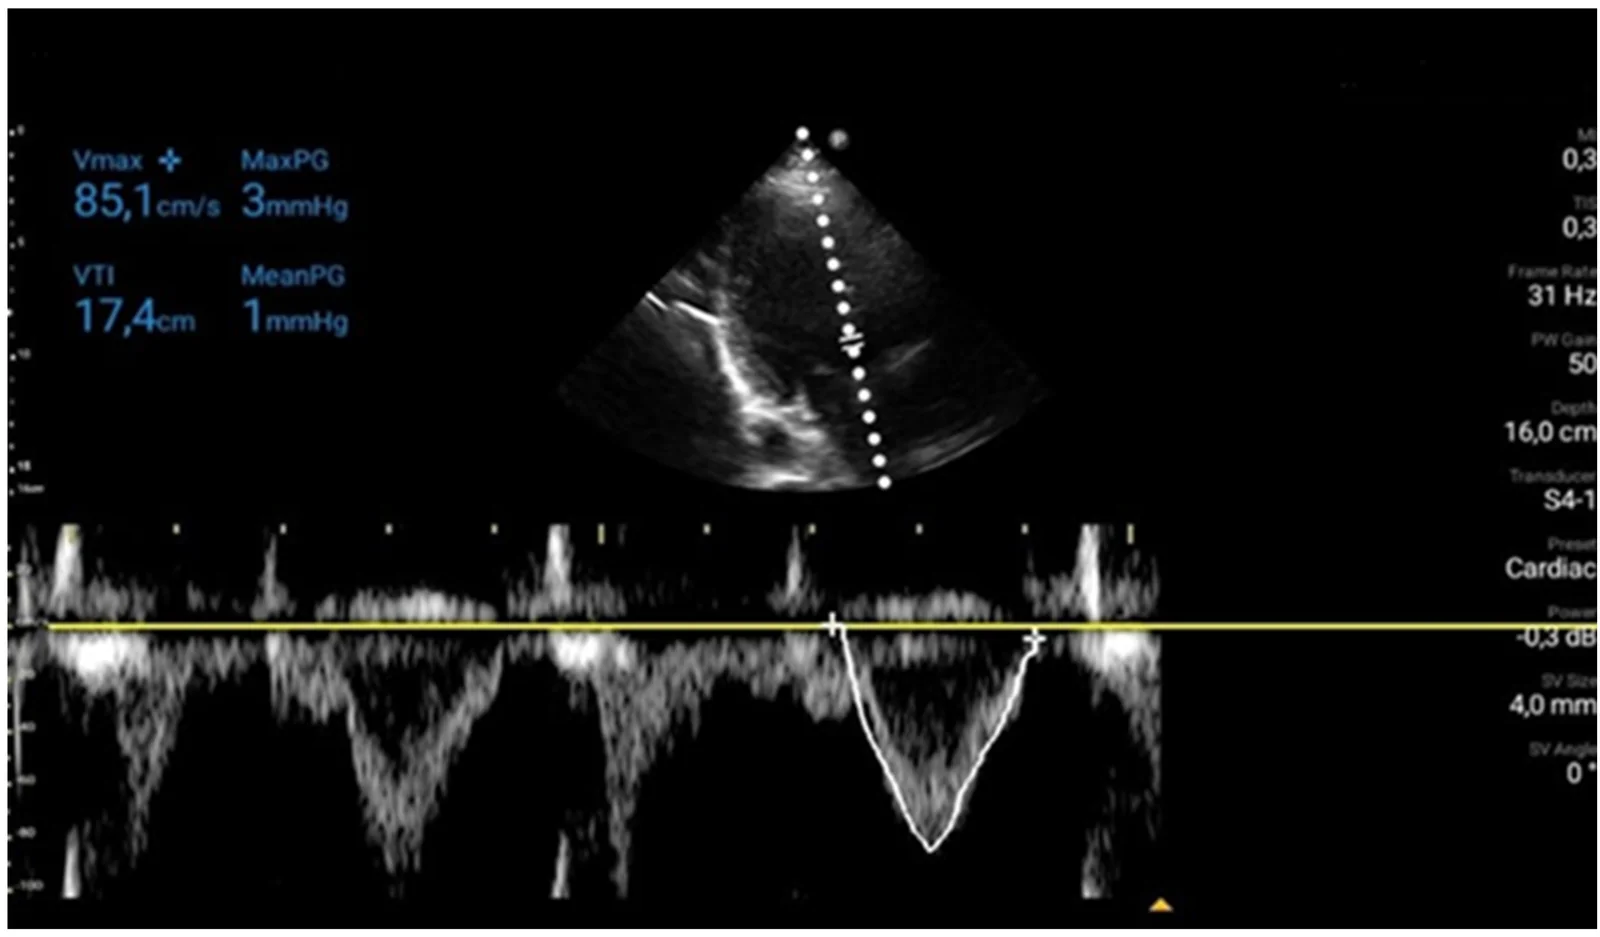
LVOT VTI measurement by pulsed-wave Doppler. Authors’ own archive. Dots line: 3–5 mm proximal of Aortic valve point, Triangle; VTI.
